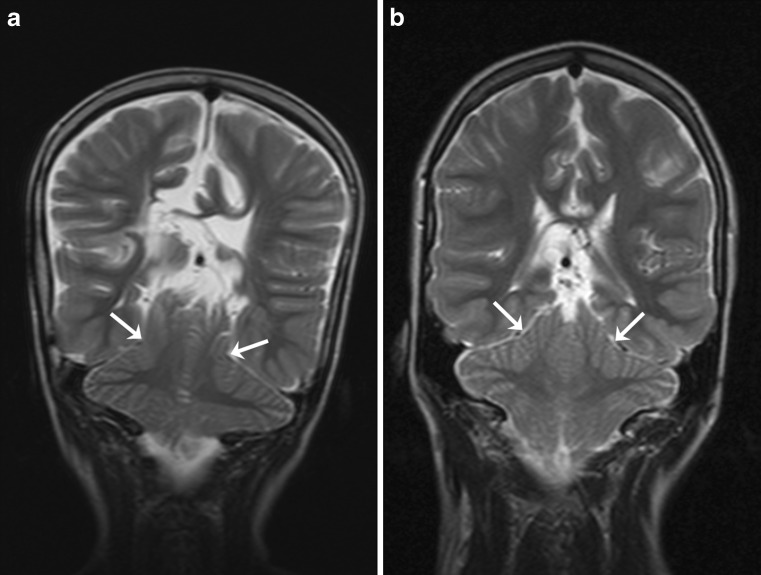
a Coronal T2-weighted brain MR image in 9-year-old child with open spinal dysraphism. The image clearly shows that the tentorium indents the cerebellar hemispheres (*white arrows*); b coronal T2-weighted brain MR image in 12-year-old child with open spinal dysraphism. In this image, it is questionable whether the tentorium indents the cerebellar hemispheres (*white arrows*)
